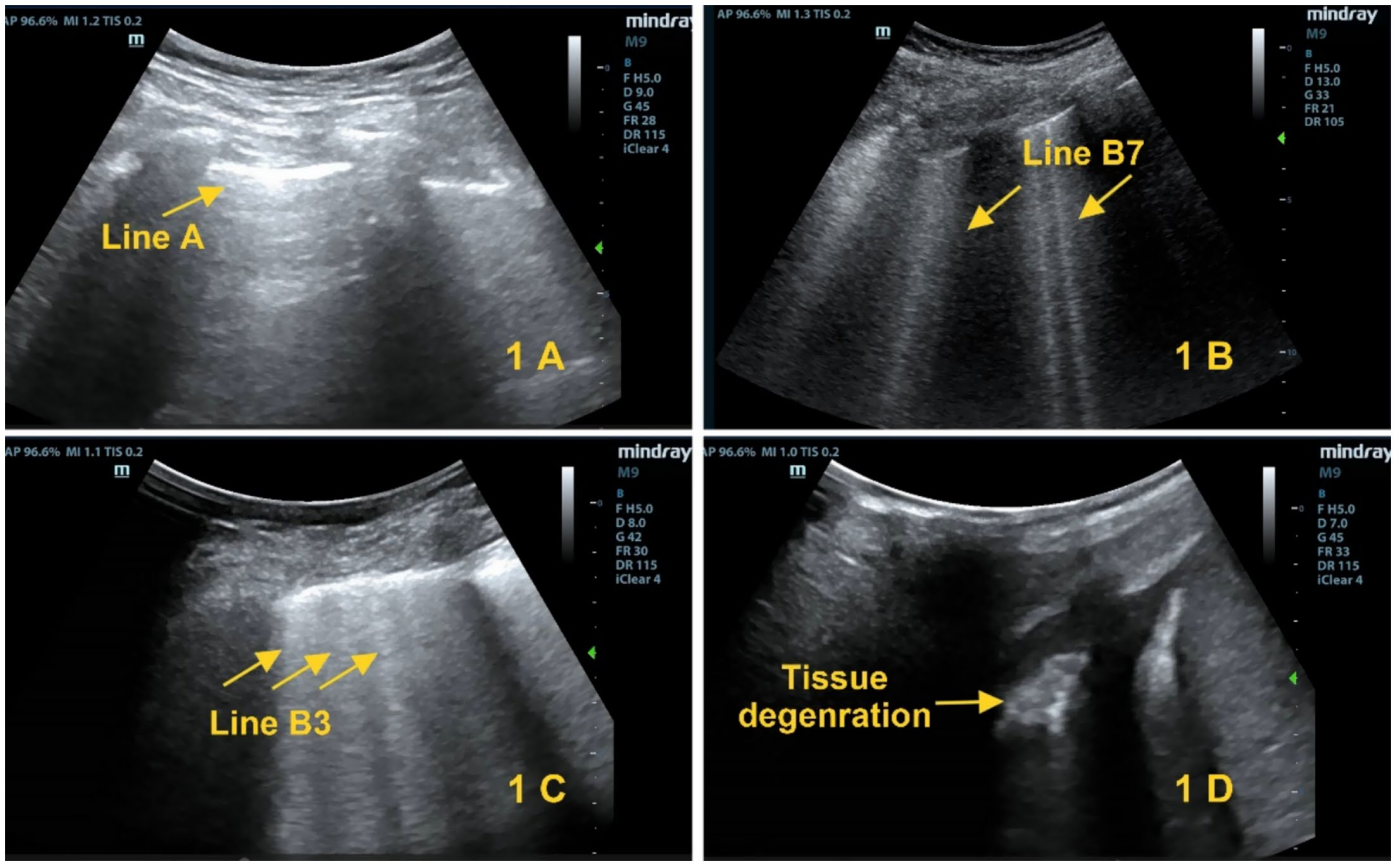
Lung ultrasound scoring criteria. (A) Score 0: A-lines (yellow arrows). (B) Score 1: B1 lines (yellow arrows). (C) Score 2: B2 lines (yellow arrows). (D) Score 3: Tissue-like sign (yellow arrows).
